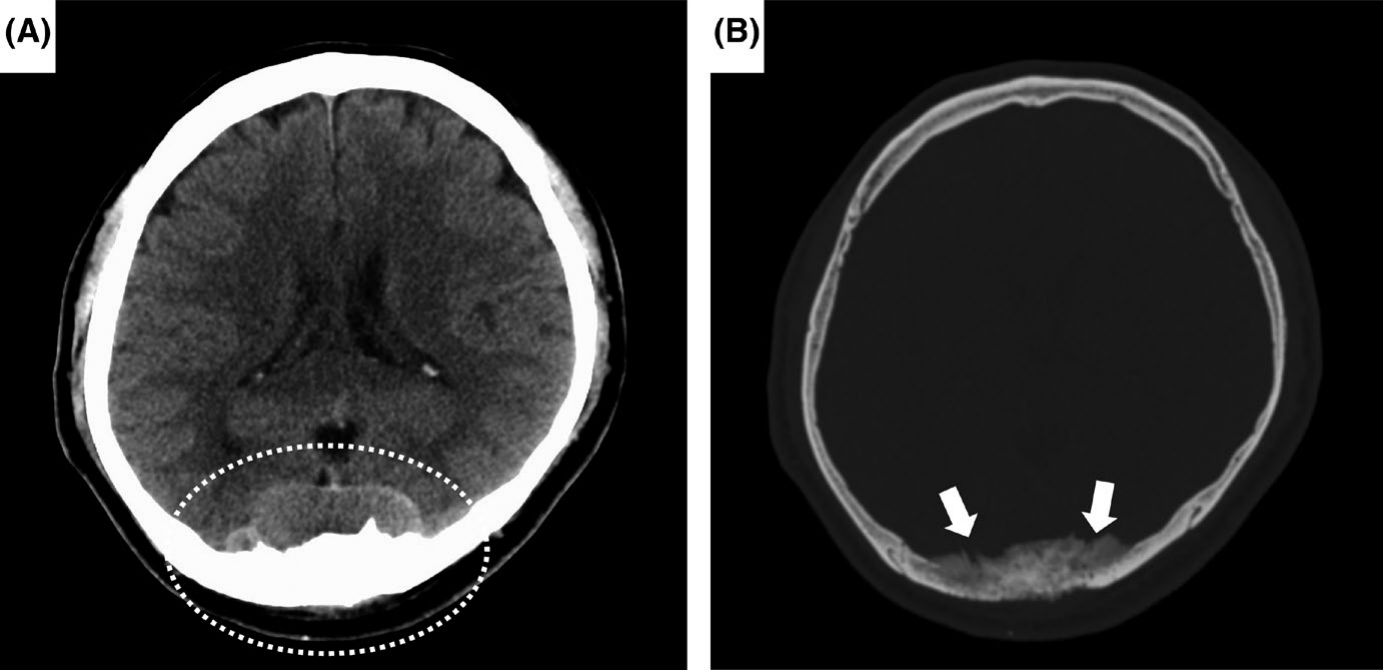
Head CT without contrast enhancement. (A) Axial brain image showing a 54 × 15 × 64 mm mass in the occipital region (A, dashed circle). The tumor is well‐defined and non‐uniform and has no internal calcification. The lesion is located in the epidural space and occipital bone and likely extends into the subdural and subarachnoid spaces. The brain is compressed; however, there are no abnormalities in the brain parenchyma. (B) Axial bone image showing thickening of the occipital bone (B, arrows). In addition, there is a bone lesion with irregular margins in which the CT value is lower than it is in the normal bone cortex
